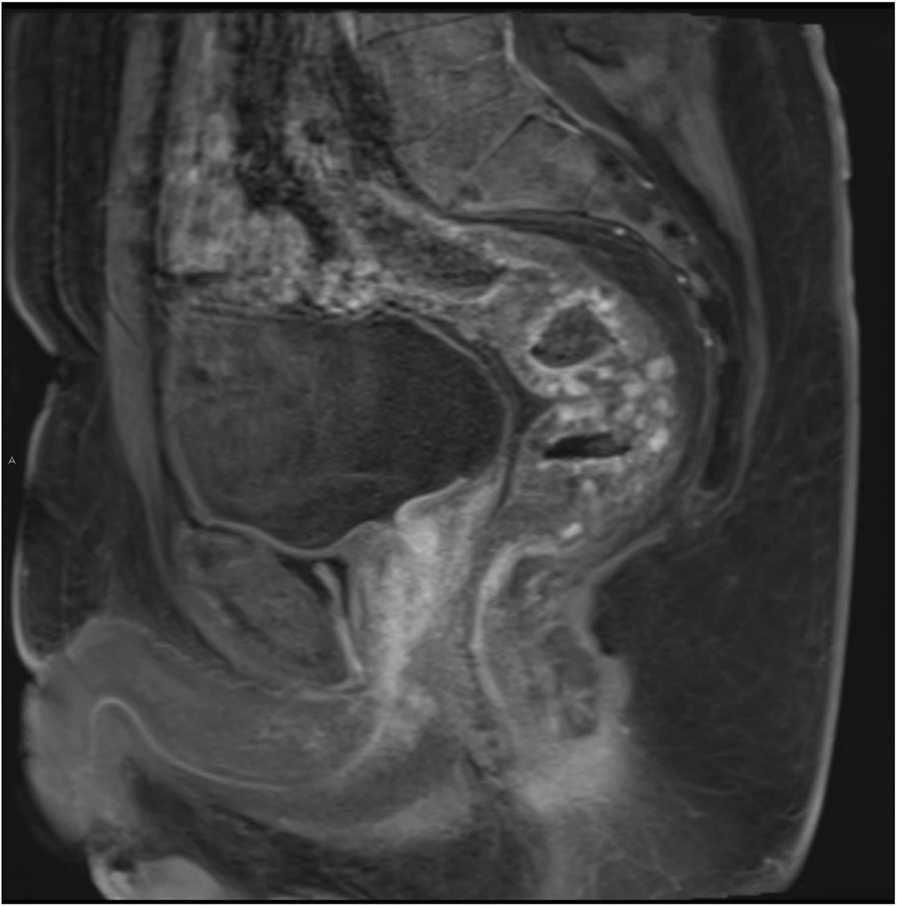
Sagittal T1 weighted MRI pelvis (early phase post-contrast fat saturated). Intense nodular enhancement of the rectal wall
